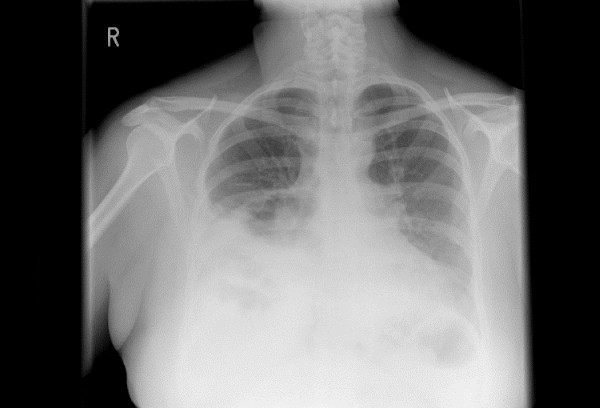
Chest radiograph (posterior-anterior and lateral view). An anterior diaphragmatic hernia containing some elements of the right colon. The heart and mediastinal contours show no gross abnormality. No obvious hilar mass. No lobar collapse or consolidation. No obvious intrapulmonary mass.
